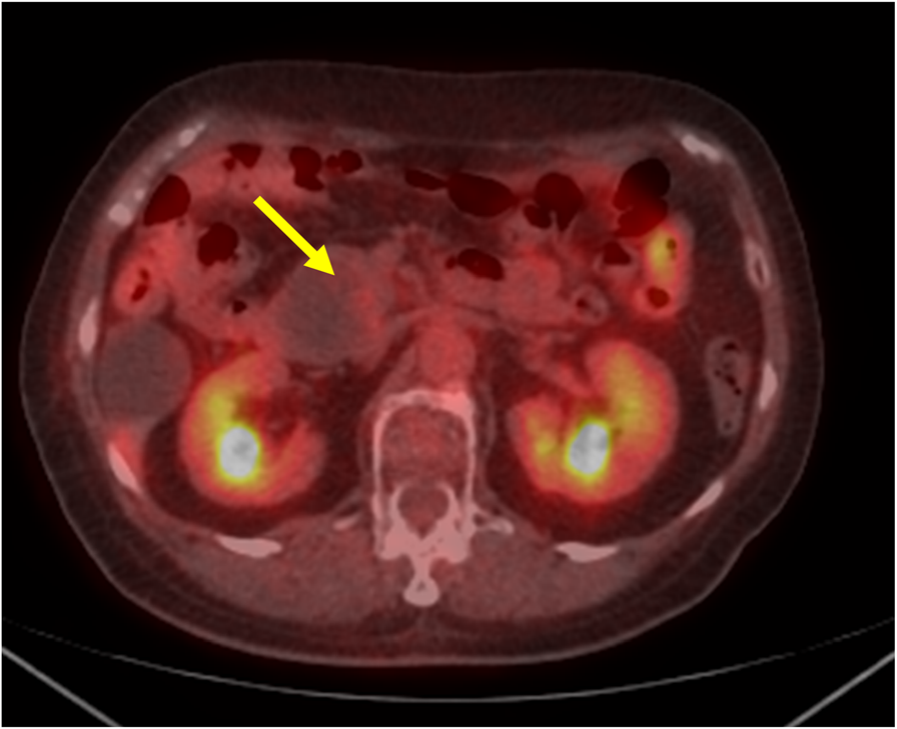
^18^F-Fluorodeoxyglucose positron-emission tomography findings (FDG-PET). FDG-PET showed FDG uptake (SUV max 1.9) in the lesion at the pancreatic head. No evidence of metastasis from other organs was observed
